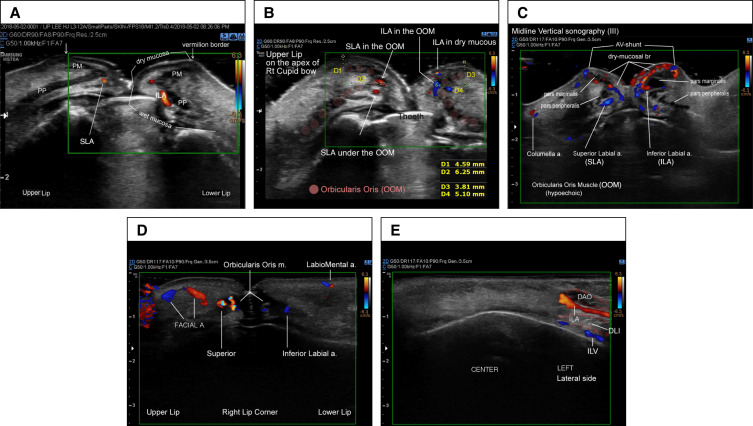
Sonographic findings. (A) Sonography at entry points 1 and 2. (B) Sonography on the vertical view of the right apex of Cupid's bow. (C) Identification of arteriovenous shunts of the labial arteries on the midline in sonography. (D) The facial artery, ILA, and SLA are located around the corners of the mouth. (E) Pathway of the inferior labial artery. DAO, depressor anguli oris; DLI, depressor labii inferioris; ILA, inferior labial artery; OOM, orbicularis oris muscle; PM, pars marginalis musculi orbicularis oris; PP, inferior labial artery; SLA, superior labial artery.
